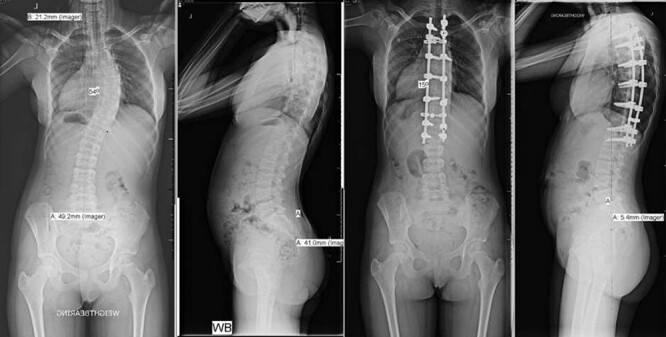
Pre- and post-operative spinal X-rays demonstrating 54° scoliosis curve, corrected with posterior fusion from T3 to L1.
